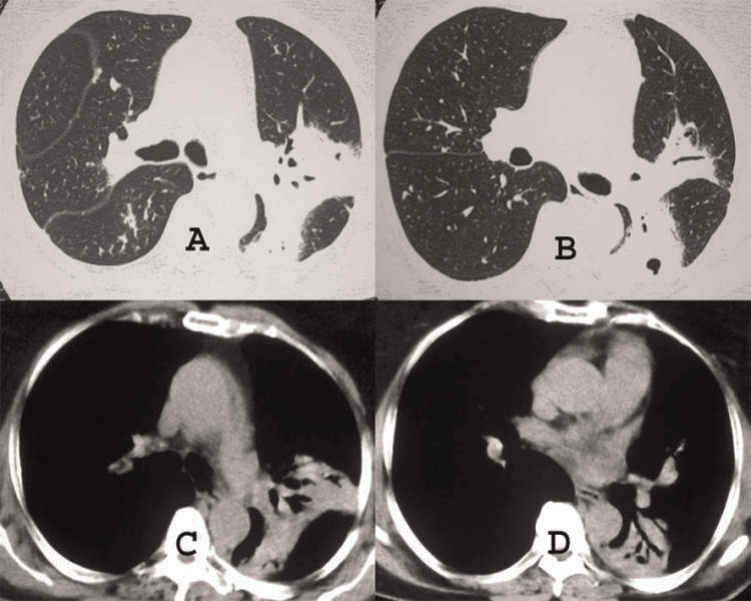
High-resolution CT scans with parenchymal (A and B) and mediastinal (C and D) window settings demonstrate a consolidation with air bronchogram in the apicoposterior segment of the left upper lobe, and in the superior segment of the left lower lobe.
